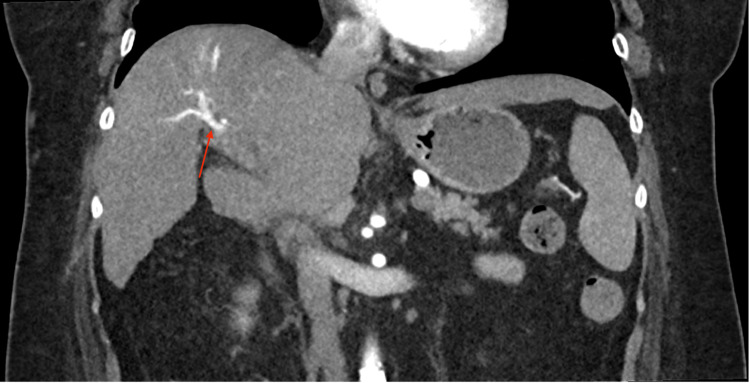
Computerized tomography (CT) scan showing thrombus extending from the liver to the heart.
